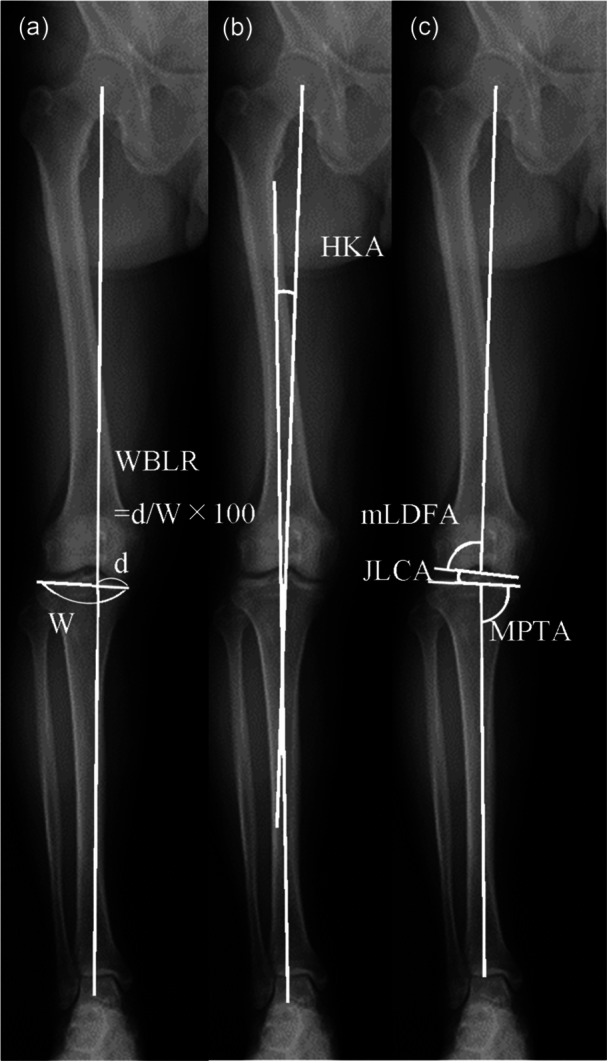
Radiographic measurements on whole‐leg weight‐bearing images. (a) Weight‐bearing line (WBL) ratio: horizontal distance from the WBL to the medial edge divided by the tibial width. (b) Hip–knee–ankle (HKA) angle: angle between the mechanical femoral and tibial axes (varus negative). (c) Medial proximal tibial angle (MPTA): medial angle between the mechanical tibial axis and tibial joint line. Mechanical lateral distal femoral angle (mLDFA): lateral angle between the mechanical femoral axis and femoral joint line. Joint line convergence angle (JLCA): angle between tangents to the femoral condyles and tibial plateau (positive values indicate lateral joint opening).
